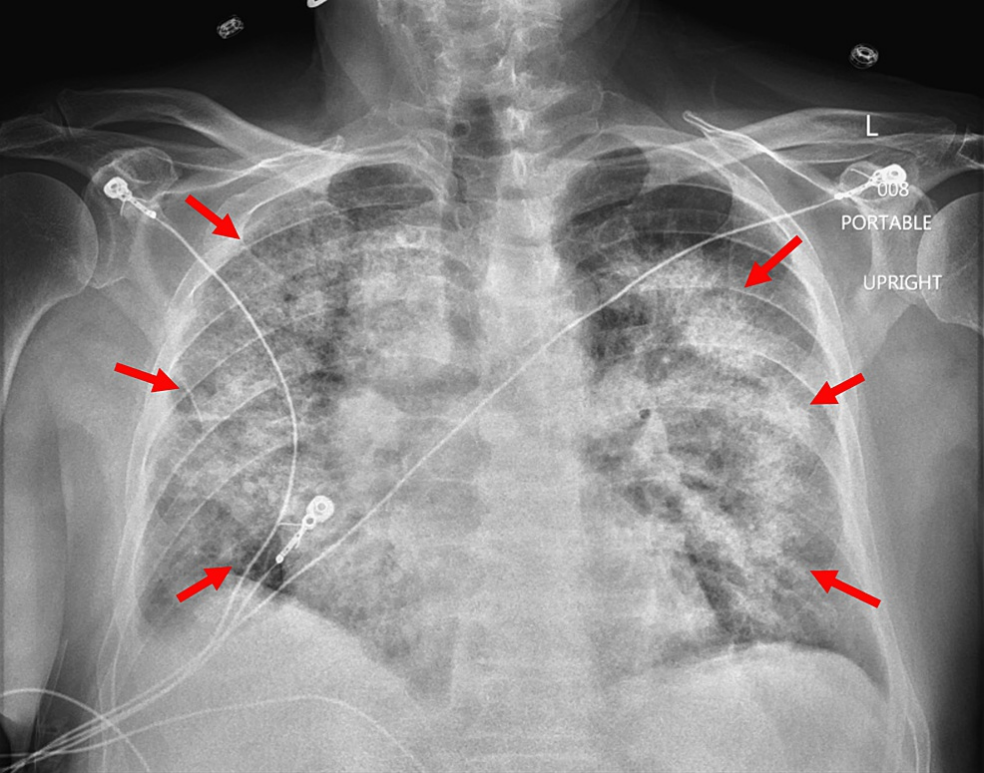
Chest X-ray demonstrating the progression of bilateral infiltrates.
